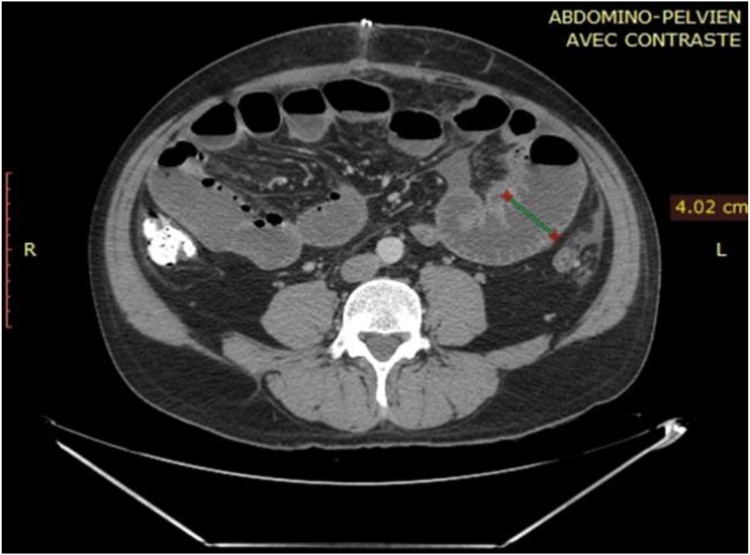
CT scan showing small bowel dilatation of 4.02 cm (green line).
